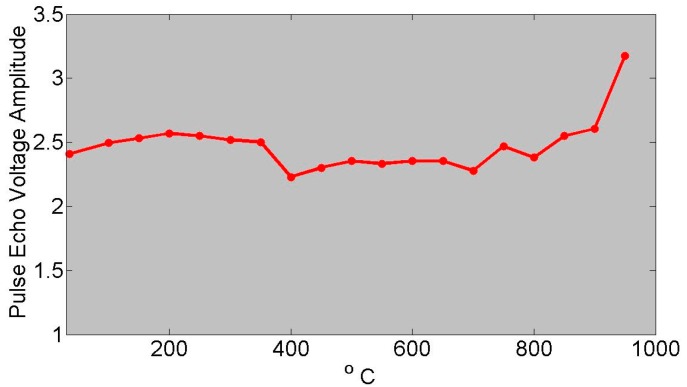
Ultrasonic high-temperature performance of single-crystal AlN wafer on steel cylinder showing acceptable performance to about 950 °C [2].
